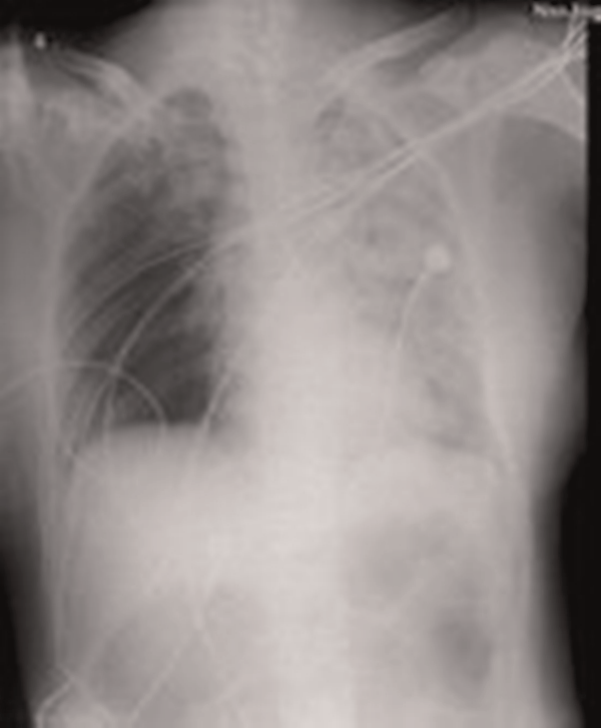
Chest X-ray film (the patient on admission of ICU).The left lung was severe compared to the right lung.
